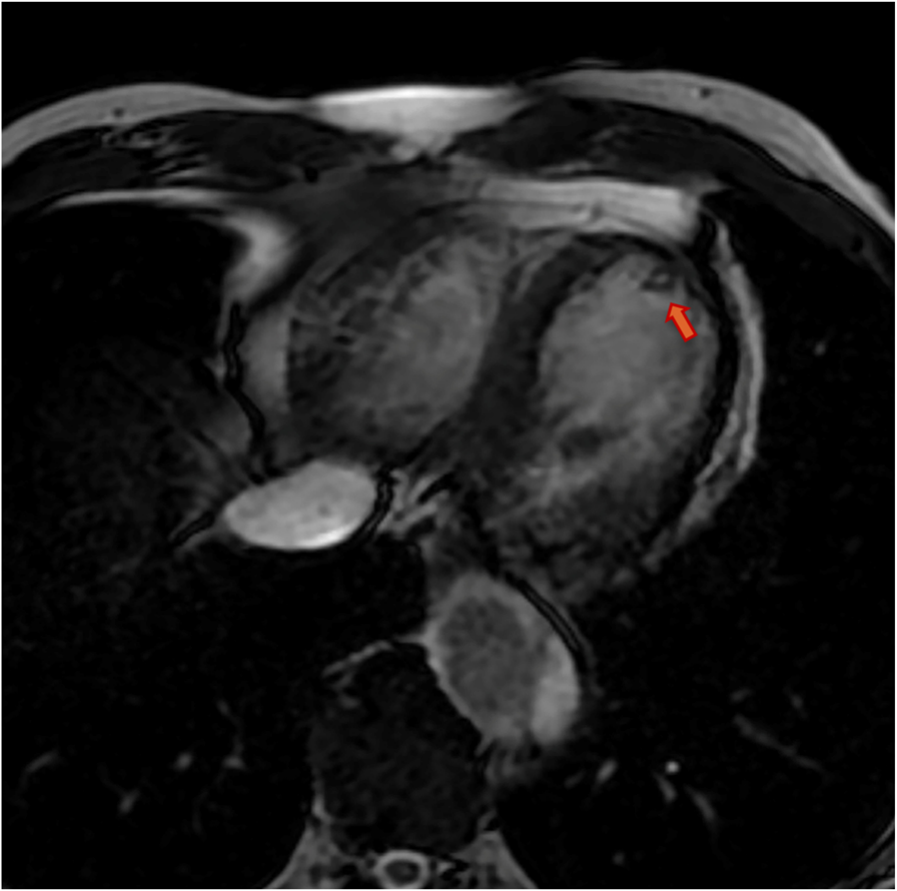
Early-enhancement cardiac MR image acquired 6 months after the acute event showing the presence of a tiny apical stratified thrombus (*orange arrow*) in the four-chamber view
